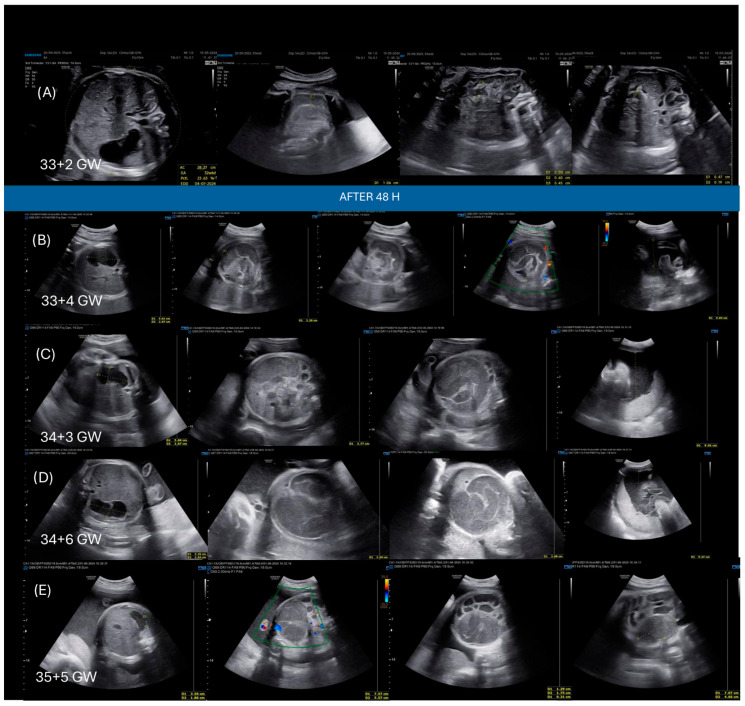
The sonographic evolution of meconium ileus complicated by intestinal volvulus in a fetus affected by cystic fibrosis. (A) Bowel dilatation of a maximum of 11 mm at 33 + 2 gestational weeks (GW). (B–D) Appearance of significant bowel dilatation with a spiral course of the loops dilated (“whirlpool sign”) with progressive increase in dilatation and appearance of polyhydramnios. (E) Appearance of meconium pseudocyst.
